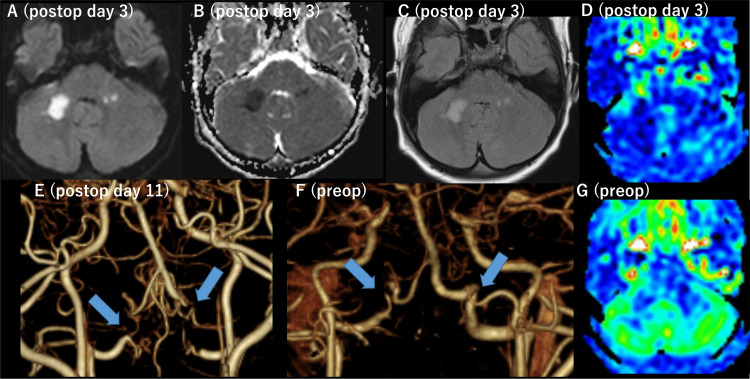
Preoperative and postoperative magnetic resonance imaging and computed tomography angiography of case 1. On postoperative day three, acute ischemic stroke was observed in bilateral middle cerebellar peduncles, appearing as high-intensity lesions on diffusion-weighted imaging (A), low-intensity lesions on apparent diffusion coefficient (B), and high-intensity lesions on fluid attenuated inversion recovery (C). Cerebral blood flow map of the arterial spin labeling method revealed diffuse hypoperfusion of the bilateral cerebellar hemispheres (D) that was not observed before the surgery (G). Postoperative CTA conducted after the onset of cerebellar ischemic stroke revealed occlusion of bilateral vertebral arteries (VAs) at the V4 segment (E, arrows) that clearly progressed from the preoperative CT angiography (F).
